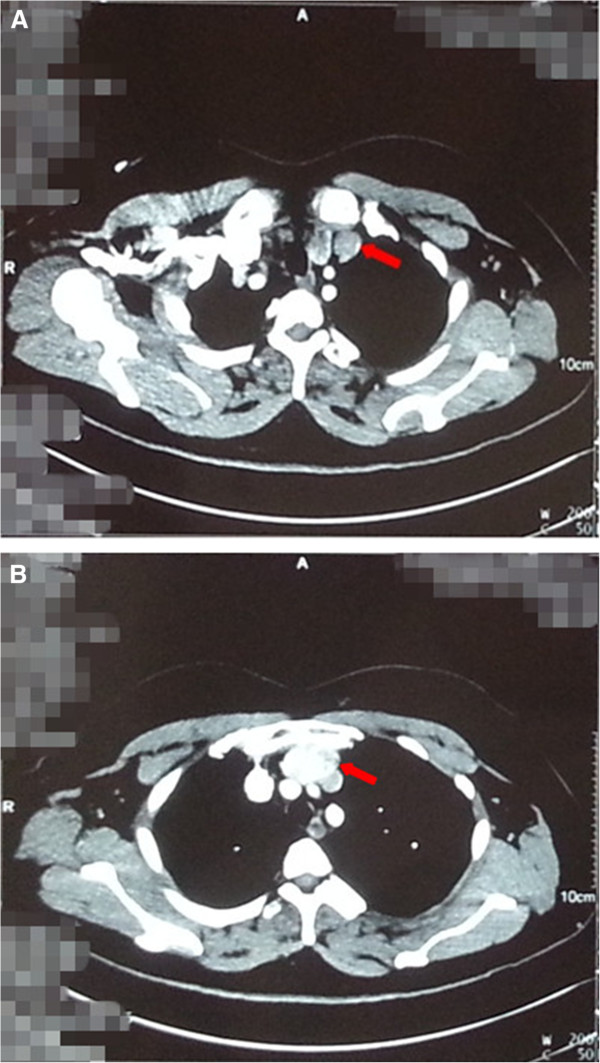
Enhanced computed tomographic scan revealed a nodule under left thyroid lobe (Figure 1A red arrow) and an anterior mediastinum mass (Figure 1B red arrow). Both the nodule and anterior mass have higher density as thyroid.
